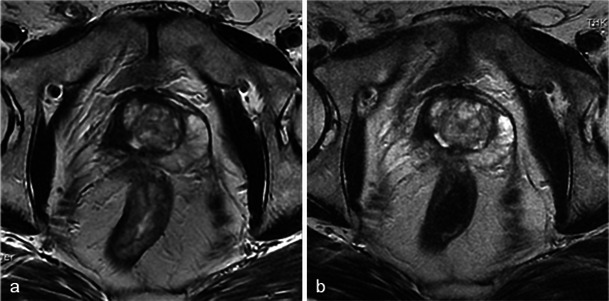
Unchanged scar appearance over time. Six-month (a) and 3-year (b) post-treatment MRI for right photodynamic therapy. The 6-month and 3 year axial T2-weighted images show the same aspect: atrophy of the right lobe with attraction of the urethra, and the disappearance of normal peripheral prostate hyperintensity. Treatment resulted in a lesser modification of the transitional zone compared with the contralateral lobe
